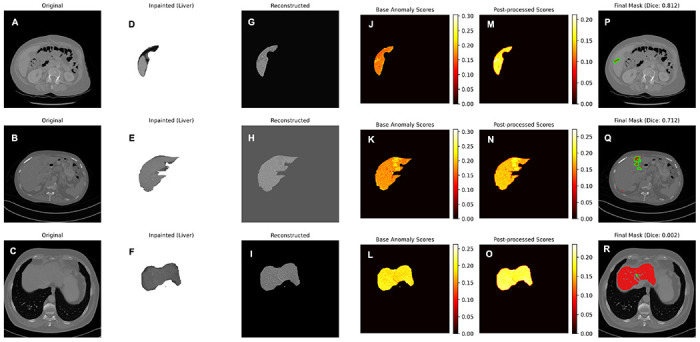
Intermediate anomaly maps across lesion difficulty tiers. Representative slices sampled from high- (top row), mid- (middle row), and low-performing (bottom row) cases (based on Dice ranks) are shown with the original CT (panels A-C), liver-inpainted input (panels D-F), encoder-decoder reconstruction (panels G-I), base (panels J-L)and post-processed anomaly maps (panels M-O), and the final prediction overlaid on the CT with the ground-truth tumor contour (panels P-R). All anomaly maps are liver-masked to emphasize organ-restricted predictions. In the final column, model predictions are shown in red, and contours, shown in green, highlight true tumor boundaries.
